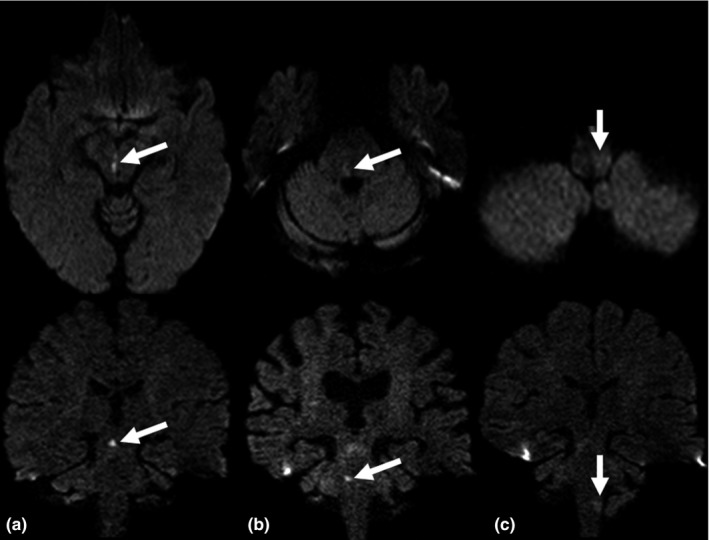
Examples of acute ischemic brainstem infarction more easily identifiable on thin‐section coronal diffusion‐weighted imaging (DWI) compared to standard axial DWI. (a) Mesencephalon. (b) Pons. (c) Medulla oblongata
